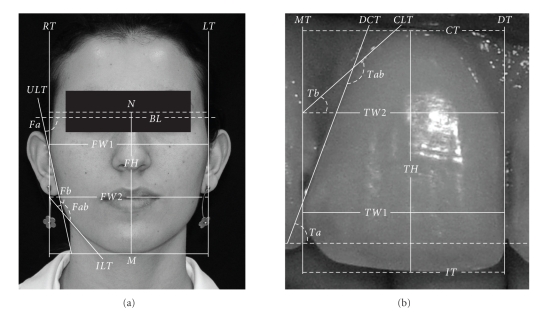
Measurements carried out to compare face and central incisors. Face: *FH*–face height, distance between nasion point (*N*) and menton base (*M*). *FW1*: maximum width of face, distance between right lateral tangent (*RT*) and left lateral tangent (*LT*). *FW2*: width of lower portion of face, at the height of the labial rima. *Fa*: angle determined by the tangent to the upper lateral face contour (*ULT*) and bi-pupillary line (*BL*). *Fb*: angle between the tangent to lateral lower contour (*ILT*) and the continuation of the line that determines *FW2*. *Fab*: angle formed by intersection of *ULT* and *ILT*. Incisor: *TH*: tooth height determined by the distance between the incisal tangent (*IT*) and the cervical tangent (*CT*). *TW1*: largest width of the incisor, distance between mesial tangent (*MT*) and distal tangent (*DT*). *TW2*: width of cervical third at the height of the limit of 1/3 of *TH*. *Ta*: angle determined by the tangent to the distal inciso-cervical contour (*CIT*) and by a virtual line parallel to the horizontal plane. *Tb*: angle formed by the tangent to the lateral cervical contour (*CLT*) and by the continuation of the line that determines *TW2*. *Tab*: angle formed by the intersection of *CIT* and *CLT*.
